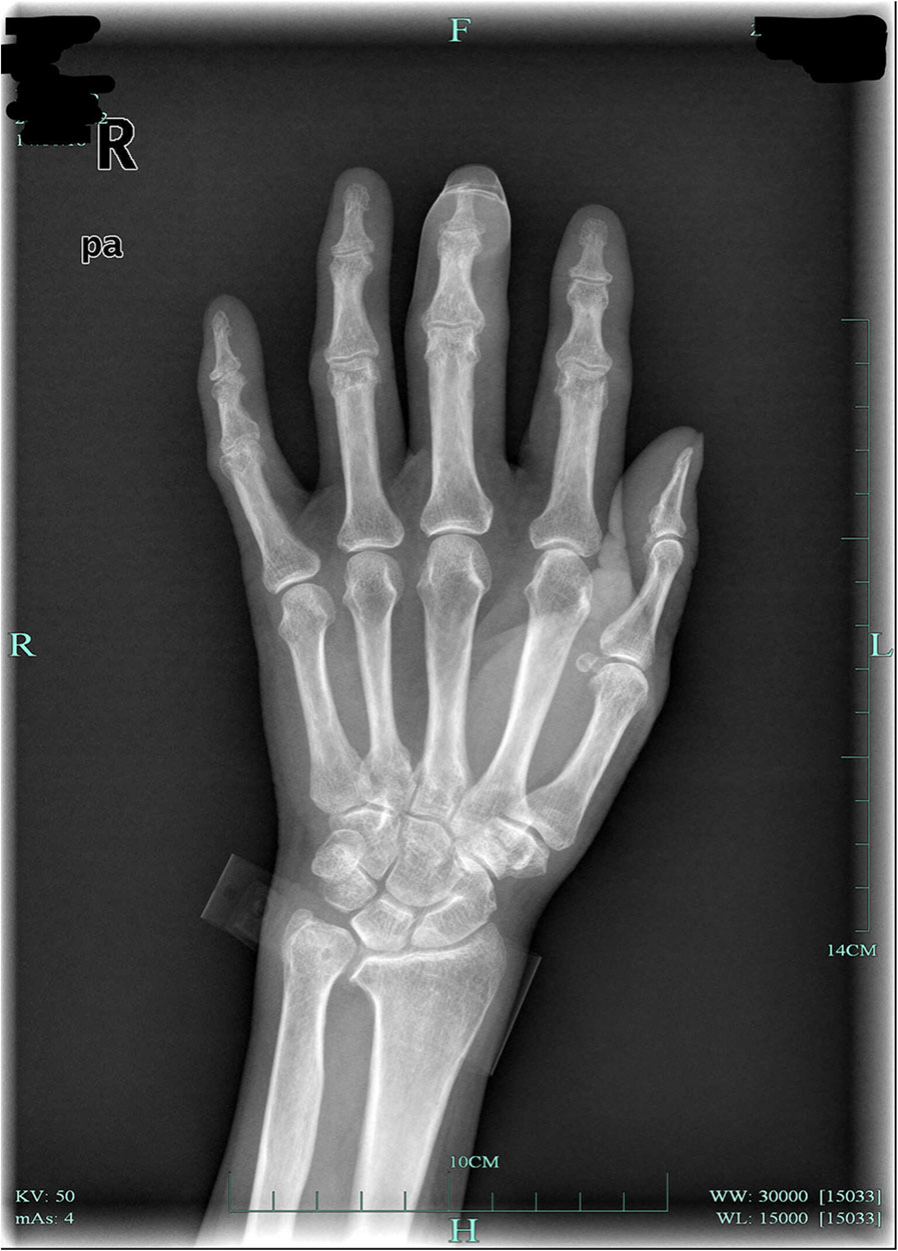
Plain X-ray films document some concavity in the distal phalanx with soft tissue swelling
